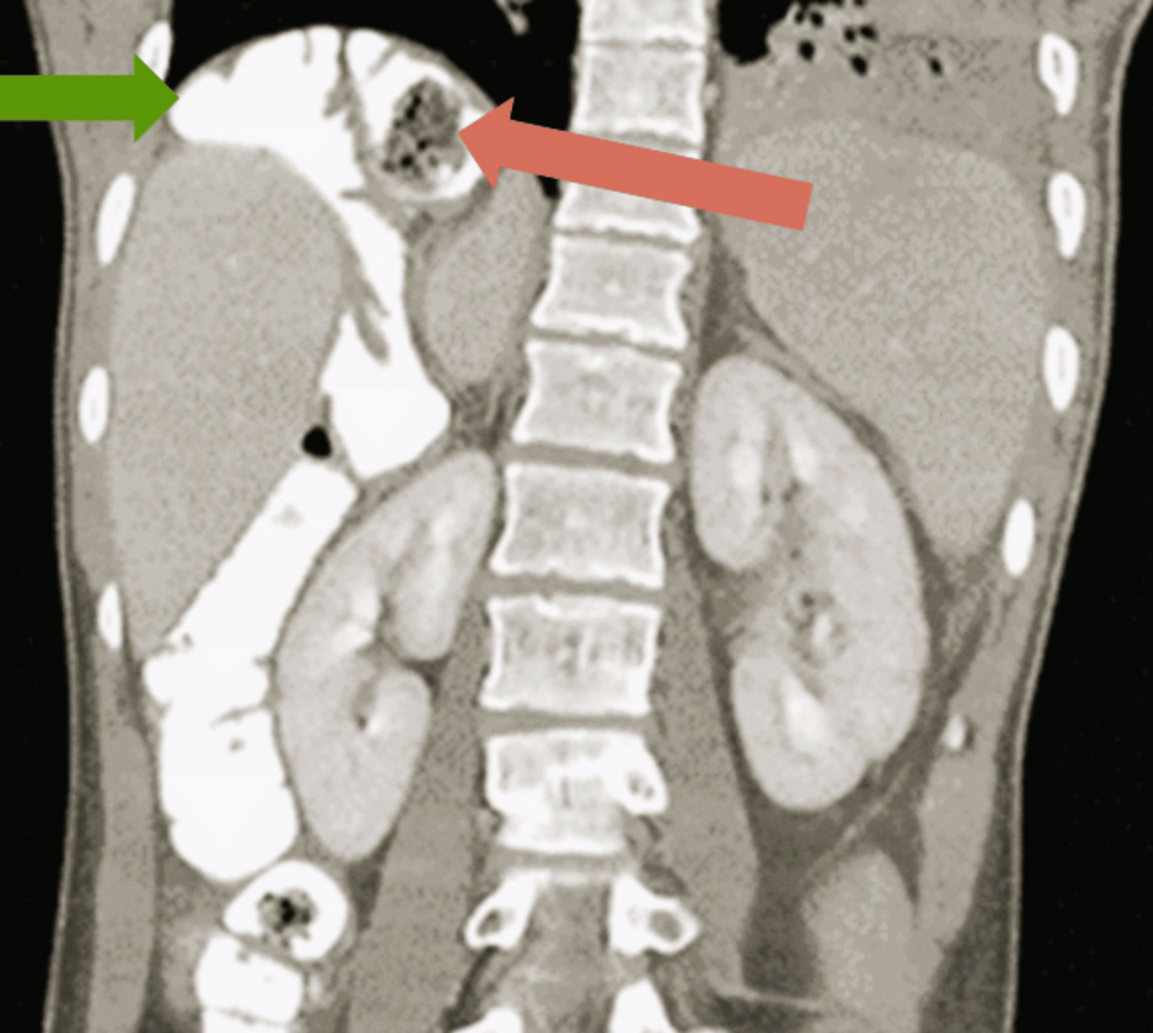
Coronal CECT image showing ascending colon between the liver and the right hemidiaphragm, as denoted by the green arrow, and the lesion, as pointed by the orange arrow CECT: contrast-enhanced computed tomography
